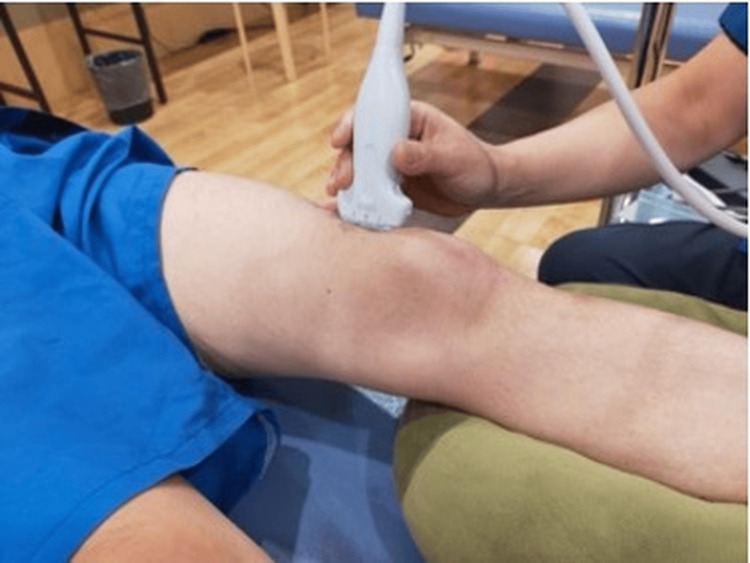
Ultrasound imaging technique of the suprapatellar pouch Ultrasound images of the suprapatellar pouch effusion were obtained by lightly placing a linear probe longitudinally on the suprapatellar pouch.
